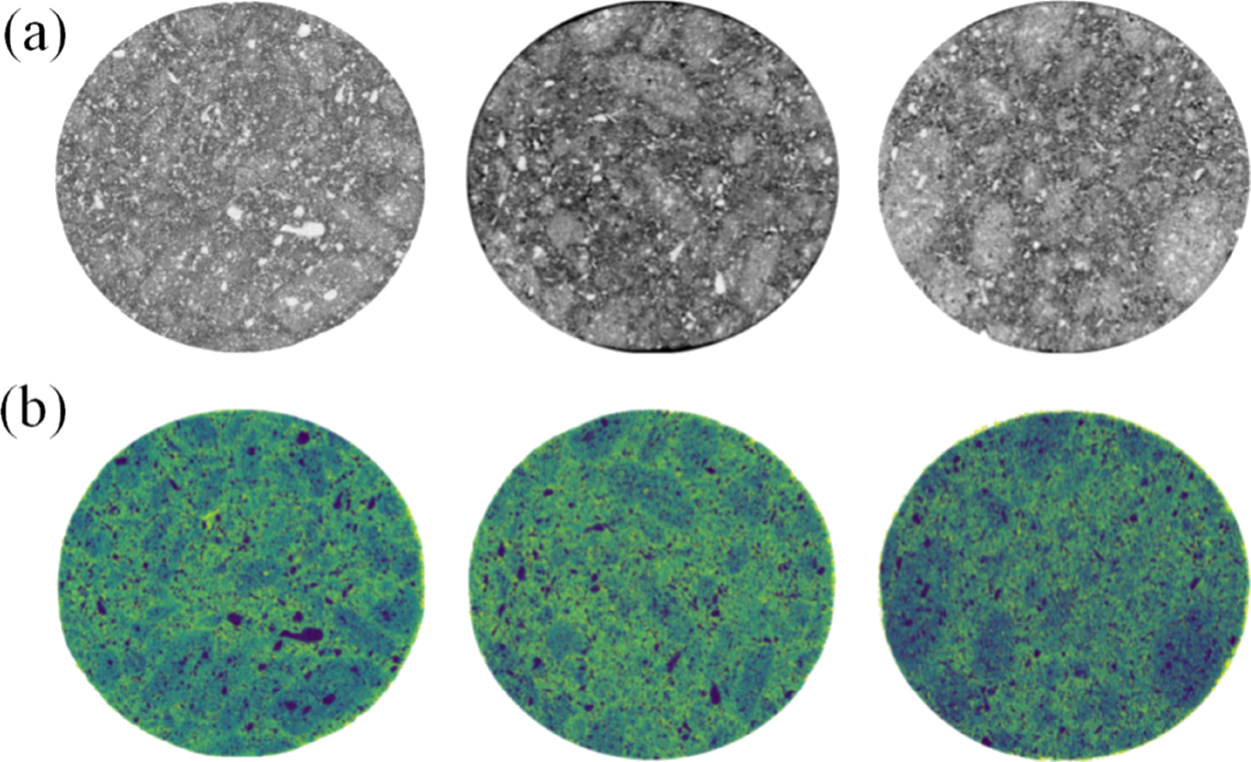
X-CT scanning pictures of cores at different positions: (a) X-CT
